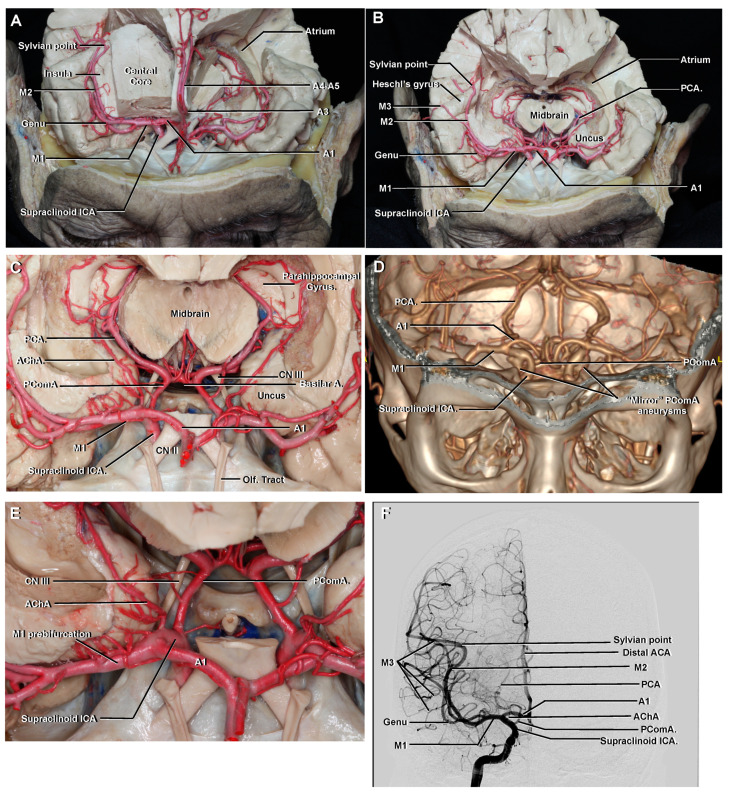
(A) Anteroposterior view. The supraclinoid ICA and its terminal branches are clearly seen. M1 originates at the ICA terminal bifurcation, courses with a horizontal trajectory and ends at the genu. M2 begins at the genu and courses with an anterior to posterior trajectory along the insular cortex. A1 courses with a horizontal trajectory to join the AComA. (B) The central core has been removed to demonstrate the arterial structures related to the mesial temporal lobe. (C) Enlarged view. The uncus and parahippocampal gyrus are limited medially by the PCA. (D) Coronal CTA VR reconstruction to be correlated with (A,C). Bilateral PComA aneurysms (“mirror aneurysms”) are seen arising immediately distal to the origin of the PComA, and they project posterolaterally towards the oculomotor nerve. (E) Enlarged view from (C). The AChA originates from the posterior surface of the ICA distal to the PComA, but proximal to the ICA bifurcation, and is closely related to the uncus. (F) Anteroposterior view of a right ICA angiogram to be correlated with (A,C). The central core of the cerebral hemisphere is located in the rectangle limited anteriorly by the A1, M1 and genu of the MCA, medially by the distal anterior cerebral artery (A2–A5 segments), laterally by the M2 segment and posteriorly by a transverse line drawn from the sylvian point.
